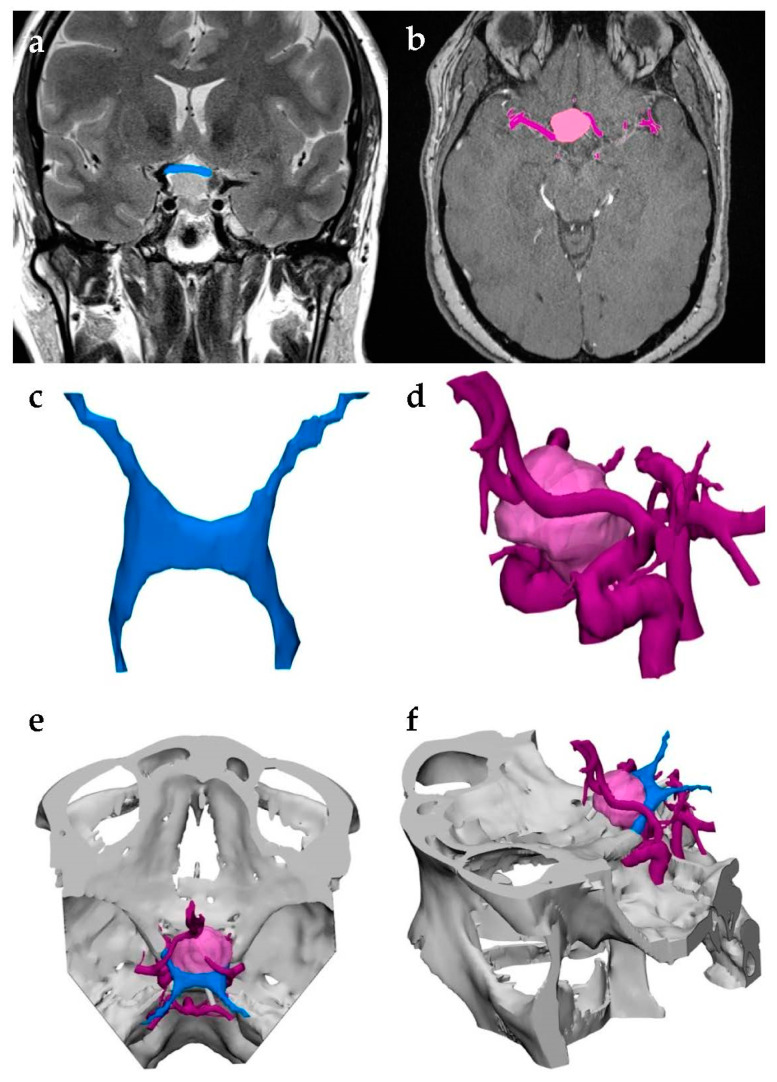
Multimodal image acquisition for three-dimensional (3D) printing. This model was used to visualize a brain tumor and distinguish it from the adjacent optic nerve and surrounding vasculature. (a) The optic nerve was captured using magnetic resonance imaging (MRI) and segmented in blue. (b) Computed tomography (CT) allowed physicians to identify the neoplasm’s borders, highlighted in pink, along with the neighboring vessels, highlighted in purple. (c,d) The segmented anatomies were converted to stereolithography (STL) files and (e,f) merged into a single model to be 3D-printed.
